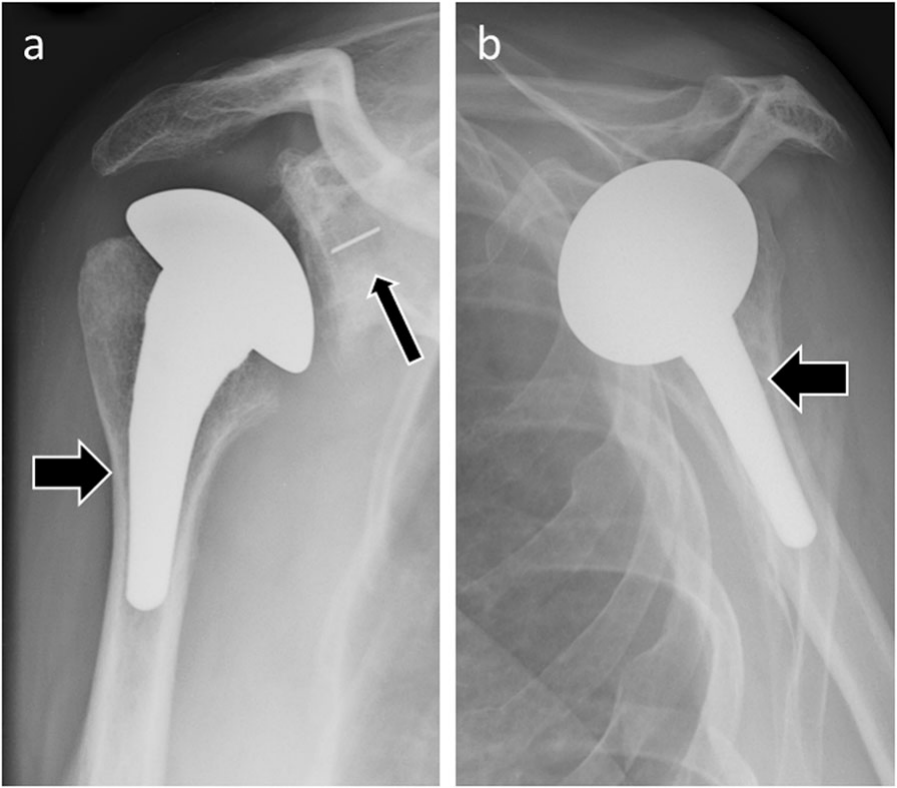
Anteroposterior (a) and scapular Y (b) radiographs of normal findings in a patient with total shoulder arthroplasty. The humeral prosthetic stem (thick arrows) is centered within the humeral shaft. The humeral prosthetic head is centered within the glenoid implant (thin arrow), with a smooth arc between the cortex of the medial humeral calcar and that of the inferior glenoid and scapular neck
